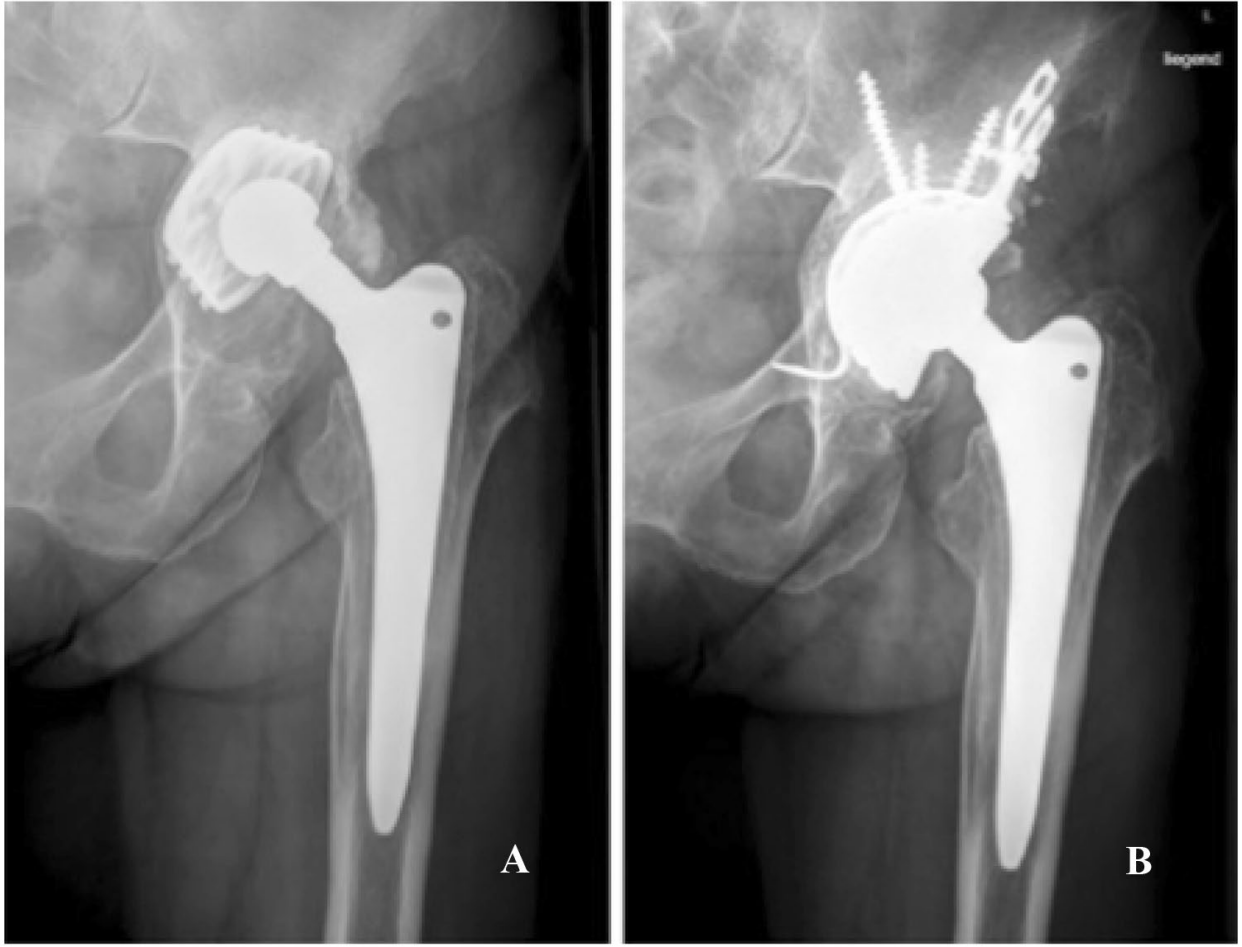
Preoperative radiograph of a Paprosky type III B defect (a). 35 months after surgery, stable position of the GAP II restoration cage and good consolidation of the bone graft can be observed (b)
